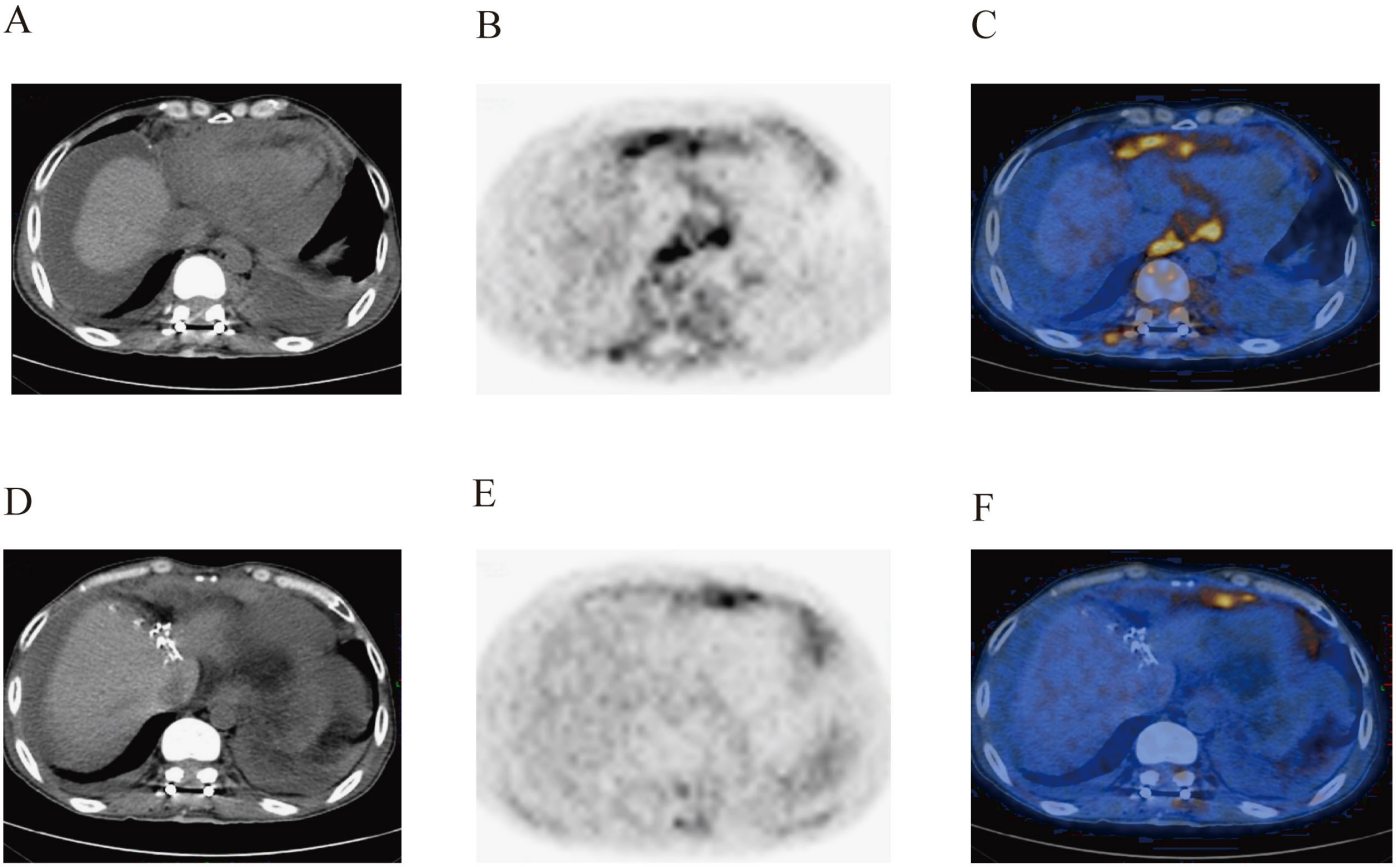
Postoperative PET-CT images of the patient’s vertebral body (A–C) and abdominal cavity (D–F). The images show the abnormal increase in metabolism in the vertebral body, paravertebral soft tissues, abdominal visceral organs, and related tissues.
